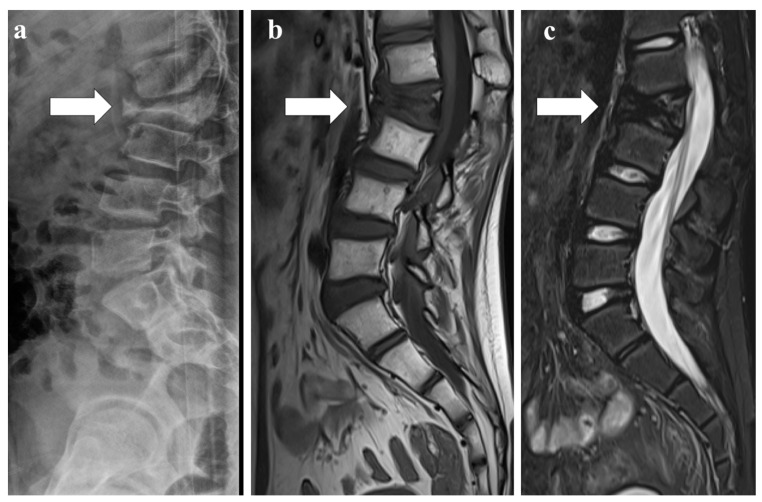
Bone metastasis from osteoblastic osteosarcoma of the extremities in a 21-year-old male. (a) lateral radiograph of the lumbar spine shows a vertebra plana in L1 with local kyphosis (white arrow). (b) sagittal enhanced T1 and (c) sagittal T2 STIR MRI confirmed the fracture with approximately 80% collapse and no signs of dural compression.
